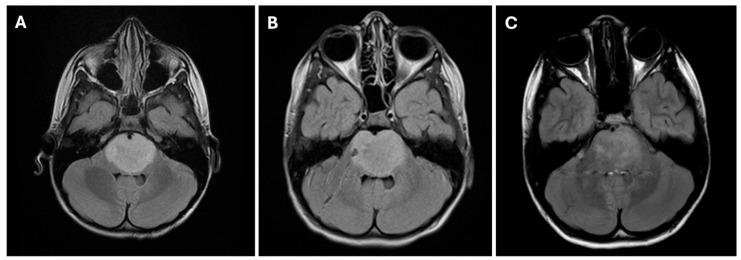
MRI of the brain of a 6-year-old boy diagnosed with DIPG prior to treatment, after biopsy, and after radiation and treatment in targeted chemotherapy clinical trial. (A) Axial FLAIR MRI brain demonstrating hyperintense expansile mass consistent with DIPG in the brainstem pons. (B) Axial FLAIR MRI brain demonstrating transcerebellar stereotactic needle biopsy track. (C) Axial FLAIR MRI brain demonstrating post-treatment reduction in hyperintensity in pons and post-treatment changes after radiation and targeted chemotherapy.
